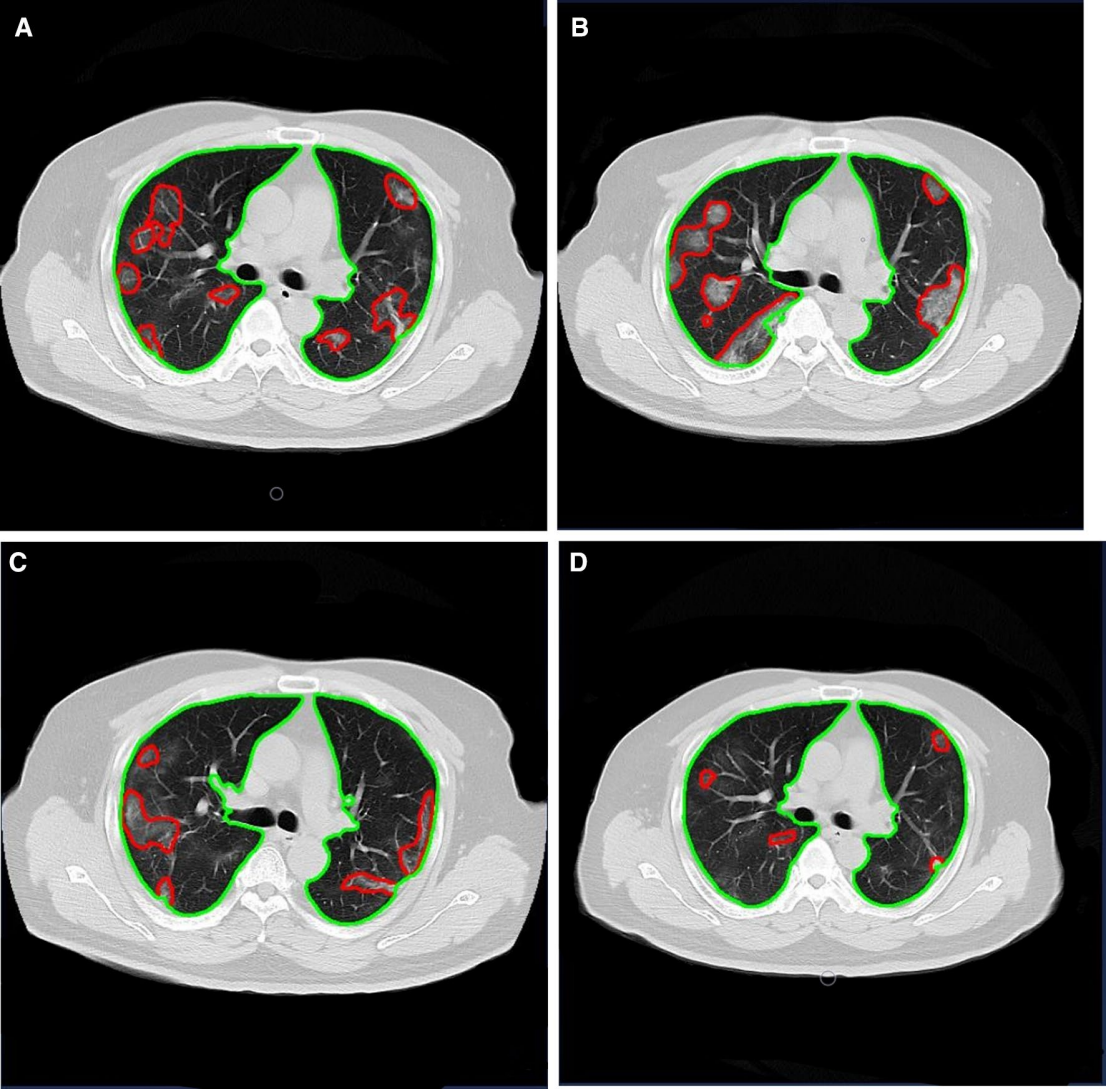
Longitudinal CT in a 41-year-old male with mild COVID-19. A Multiple mixed GGO in both lungs under the pleura. B The size and number of GGO increased. C GGO slightly absorbed compared to the second follow-up. D Residual GGO in both lungs were seen in the third follow-up
